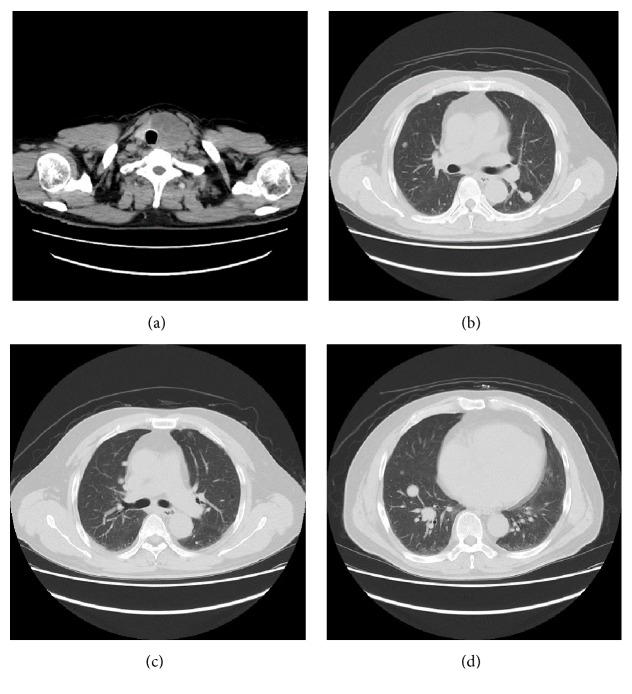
(a–d) Computed tomography showed tumor with eggshell calcification of the thyroid left lobe and multiple lung metastases. There were no bulla and bleb.
